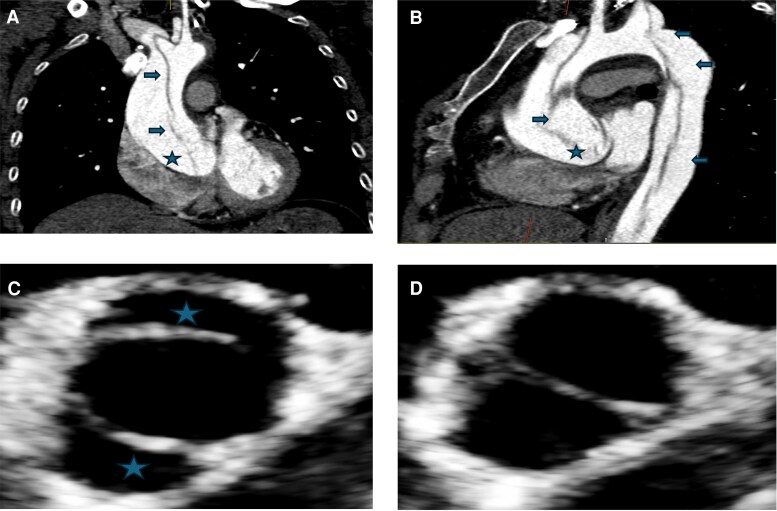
Cardiac imaging in Turner syndrome. A and B, Computed tomography scans of ascending and descending aorta, demonstrating dilated ascending aorta with dissection flap, marked with arrow extending from dilated aortic root, marked with star, through ascending aorta to descending aorta. C and D, Transthoracic echo demonstrating type 1 bicuspid aortic valve; panel C is open valve and panel D is closed valve. Anterior-posterior leaflets are marked with a star.
